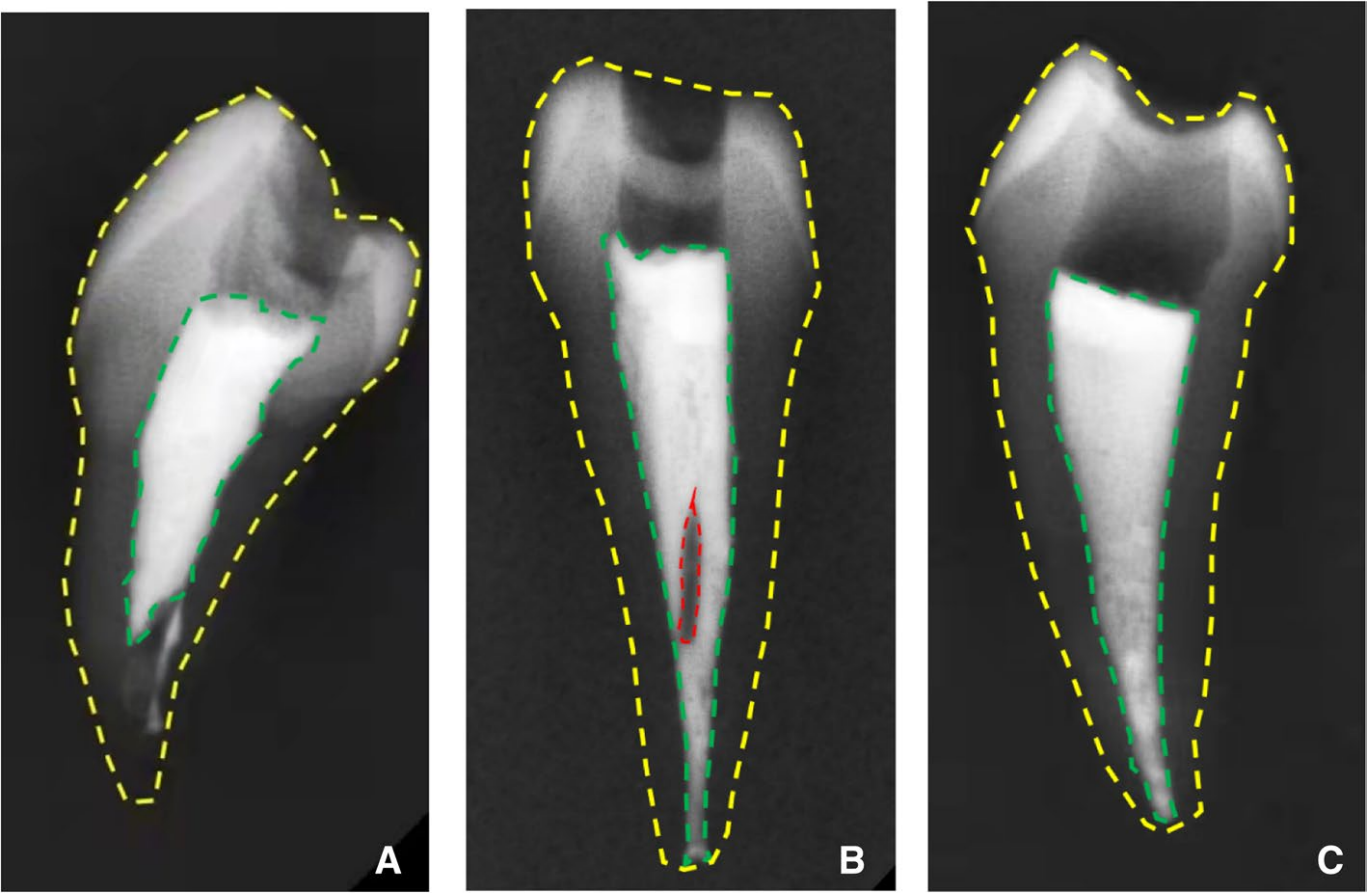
X-ray images of three typical samples. The tooth profile was outlined by a yellow dotted line. The root filling was outlined by a green dotted line. And the void was outlined by a red dotted line. A A root canal filled by a student from LG in test was considered unaccepted in terms of filling length. B A root canal filled by a student from LG in test was considered unaccepted due to void presence. C A root canal filled by a student from WFG in test was considered to have adequate root length and no void
